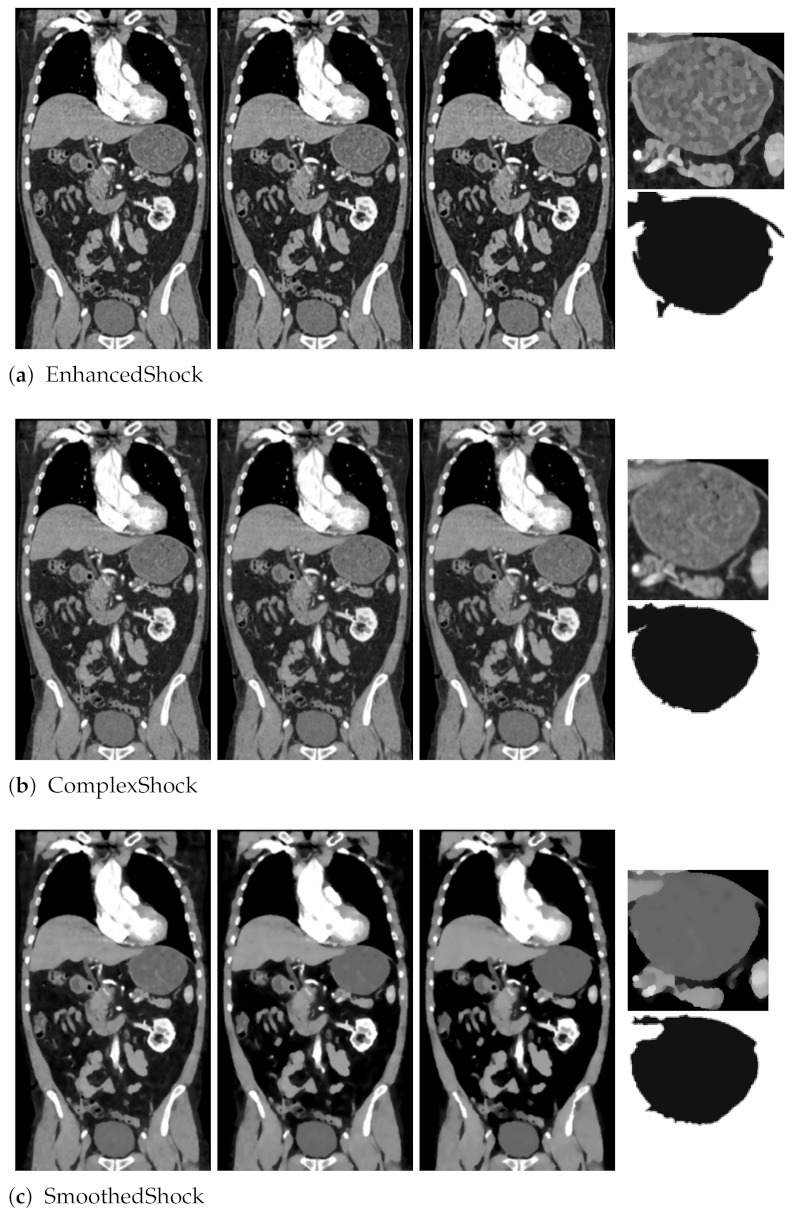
Segmentation obtained from one CT slice, with different methods, after 10, 20, and 30 iterations. A part of the segmentation is also presented.
